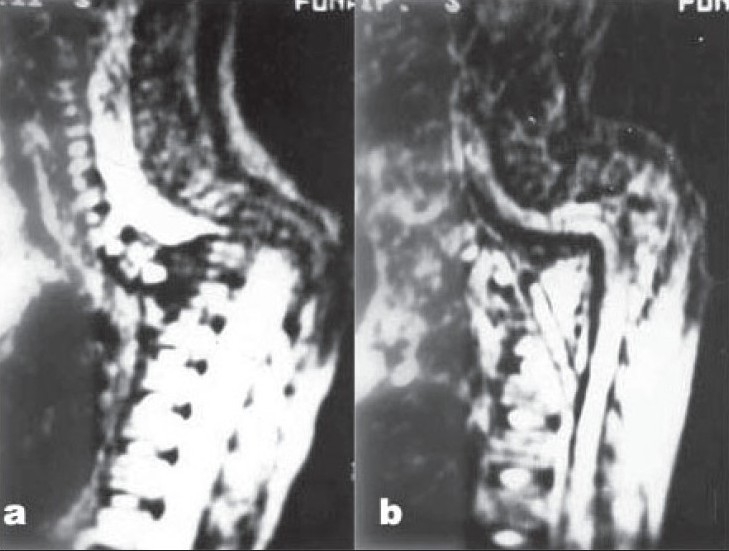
(a) Mid sagittal T2 WI MRI of upper dorsal spine showing forward tilt of the C7 body over fused posterior cartilaginous remains of D1-D3 vertebral bodies and D4 vertebral body causing severe compression of cord at D4 body. (b) Postoperative mid sagittal T2 WI MRI showing through decompression of the cord and fibular graft bridging the gap. note the organized soft tissue between graft and cord.
